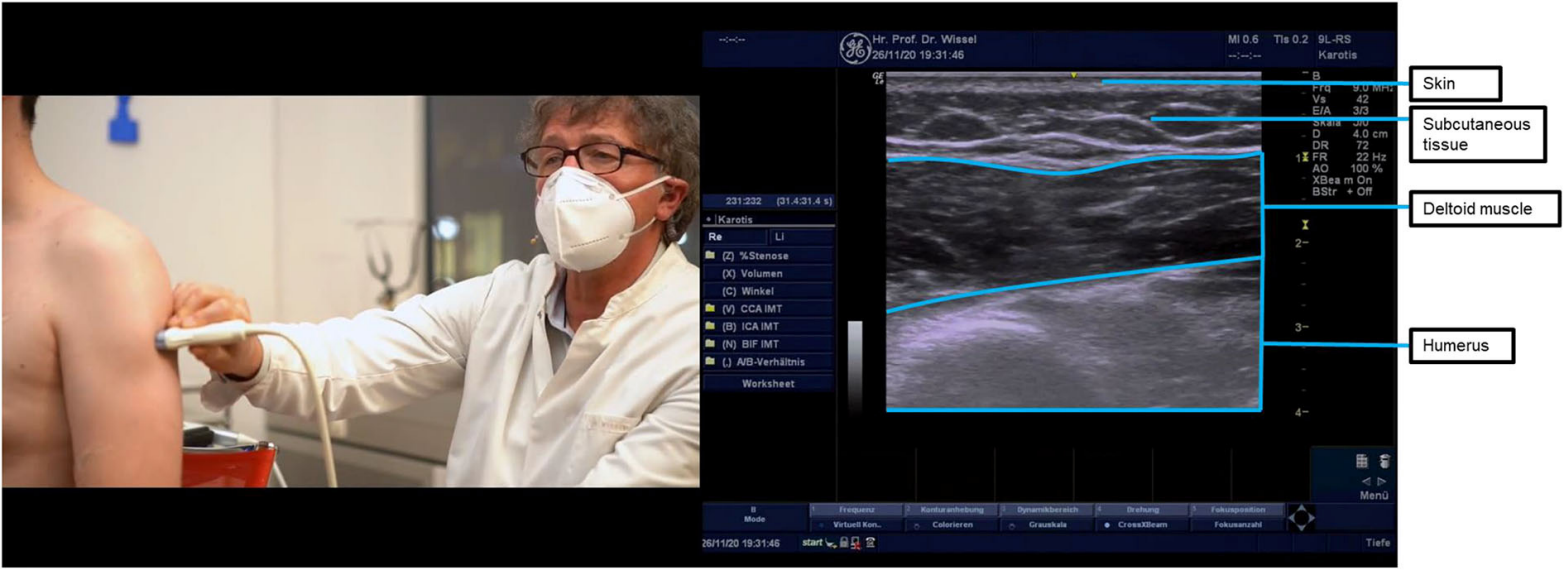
Ultrasound probe placement and image of the intermediate deltoid (pars acromialis) muscle. The deltoid has a monomorphic appearance with stripes, when the ultrasound probe is placed longitudinally, and dots with facias/tendon-like structures, when the probe is transversally orientated. The injection target to treat retroversion and extension is the posterior part of the deltoid muscle. In the figure, the probe is compressing the arm to show the deltoid muscle at the top and humerus muscle at the bottom of the ultrasound image. To visualize and inject the anterior deltoid, the probe should be slid anteriorly from the position shown; to visualize and inject the posterior deltoid, the probe should be slid posteriorly.
